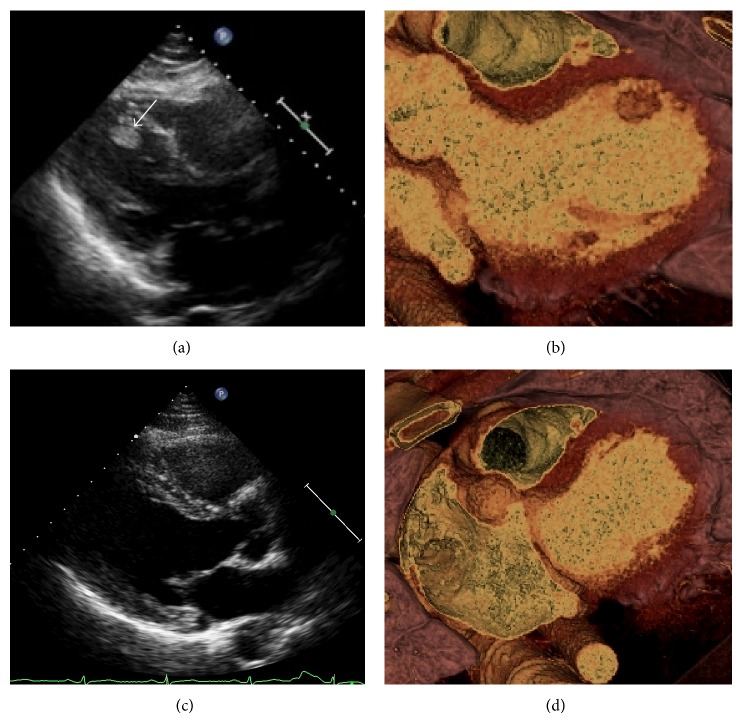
Imaging findings of a ball mass in the left ventricle (LV) on admission. This imaging revealed a ball mass (arrow) in the left ventricle (LV) detected by transthoracic echocardiography (TTE) (a) and contrast enhanced computed tomography (CT) (b). We confirmed that it had disappeared after anticoagulant therapy including rivaroxaban on the 14th day from TTE imaging (c) as well as CT imaging (d). Therefore, we diagnosed this ball mass as a thrombus.
